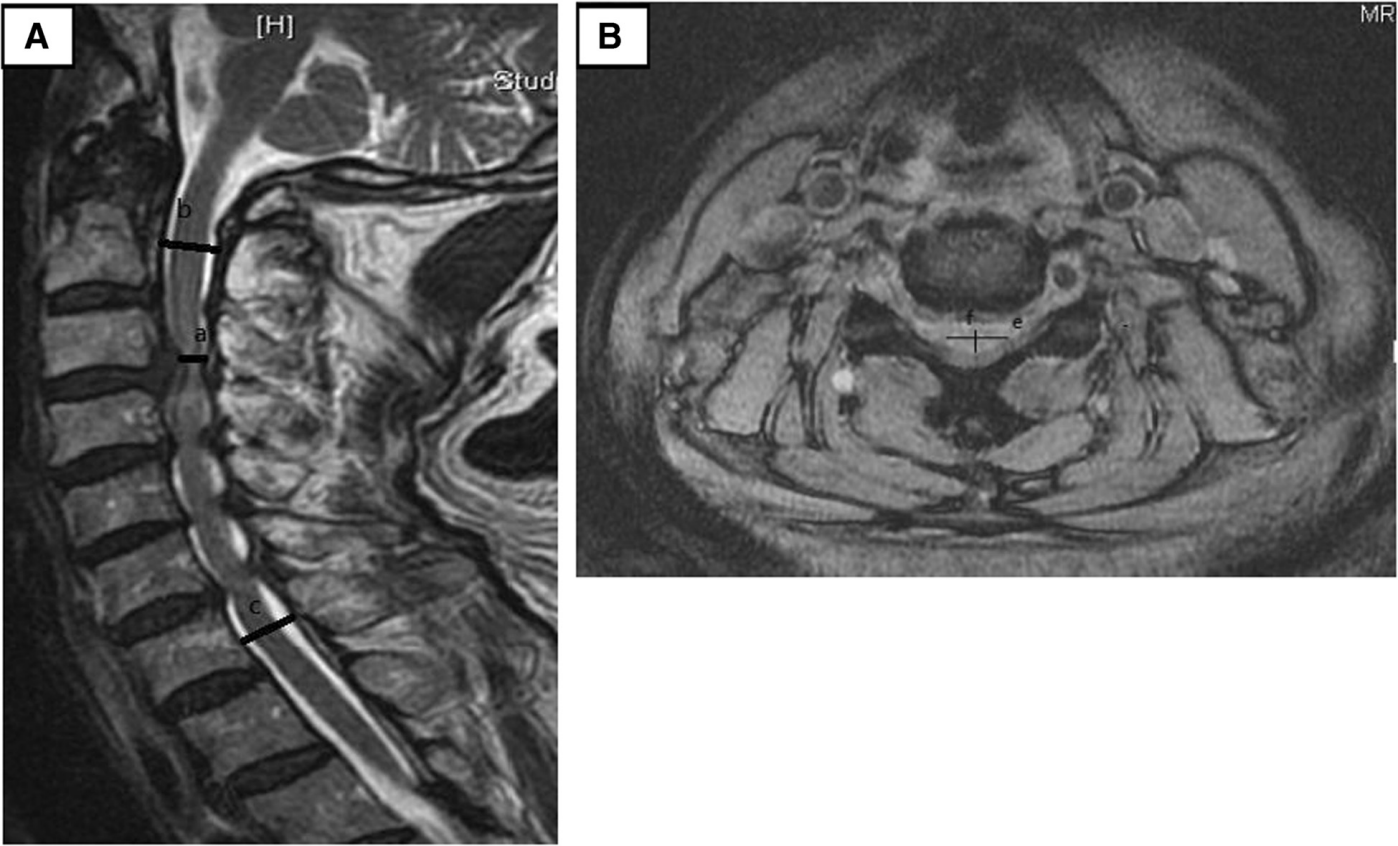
T2 MRI image of cervical spine. (A) Sagittal view. Sagittal compressive ratio = *a* × 2/(*b* + *c*). (B) Axial view. Axial compressive ratio = *f*/*e*.
